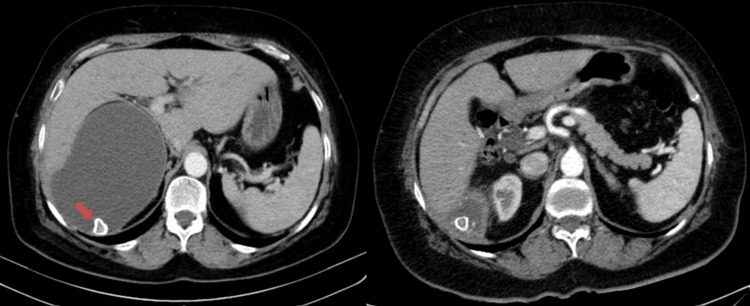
Axial CT image shows a foreign body (calculi) with calcified periphery (left, arrow) and a consequent reduction in the size of the fluid collection located in the hepatorenal space (right).
